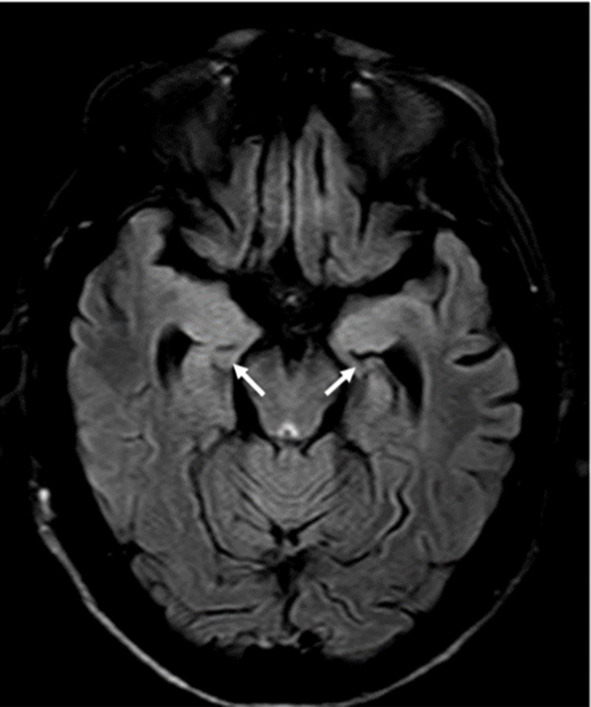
Brain MRI FLAIR protocol, showing signal hyperintensity, and atrophy at the mesial temporal level and bilateral hippocampi.
